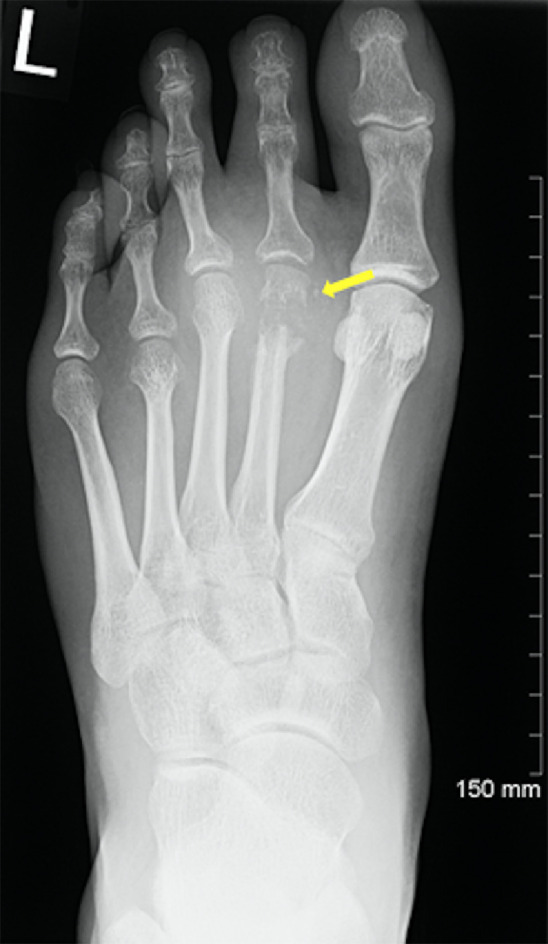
Oblique and DP radiographs of the left foot from Feb 2020, 6 months post initial presentation. There is marked expansile metaphyseal and cortical erosion centered on the neck of the second metatarsal (arrows). There is associated soft tissue swelling with some internal calcification.
